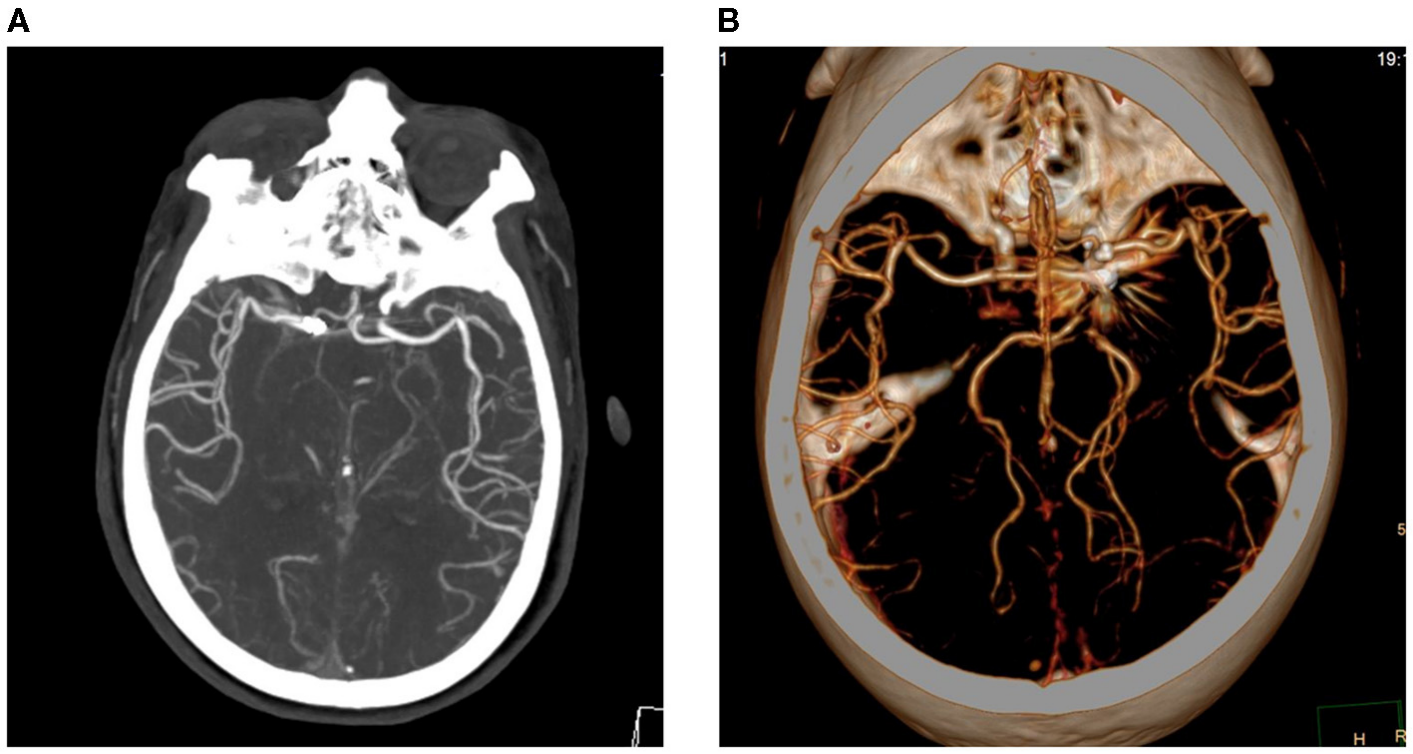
CTA performed 2 hours later indicated no occlusion of the right ICA (A,B).
